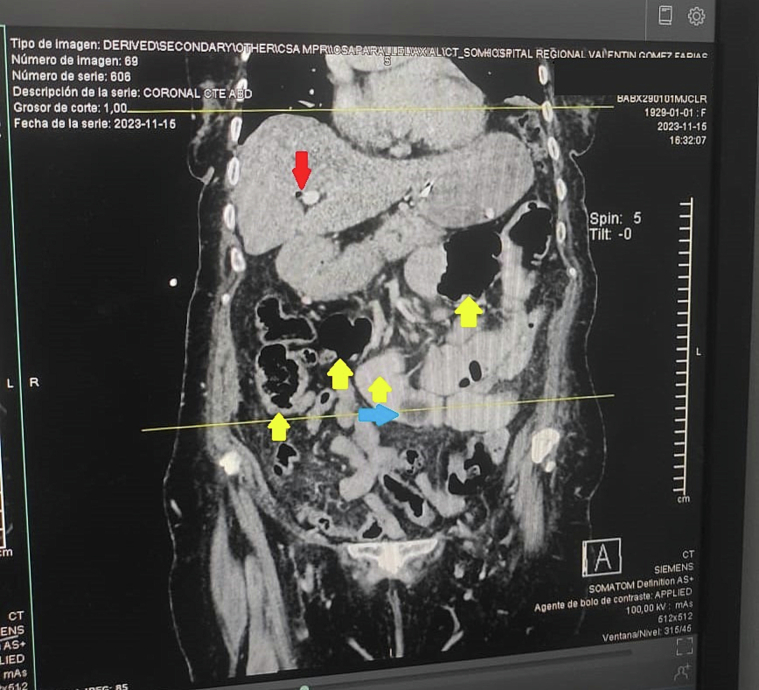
Shows a coronal view of a computed tomography scan of the abdomen, revealing multiple distended intestinal loops, suggestive of an intestinal obstruction. Additionally, pneumobilia is clearly visible, which is a key finding in the diagnosis of biliary ileus. These features are consistent with Rigler's triad, which includes intestinal obstruction, pneumobilia, and the presence of an ectopic gallstone. Red Arrow: Indicates pneumobilia, which is the presence of air within the biliary tract. Air should not be present in this location unless the patient has undergone an endoscopic procedure, such as an ERCP, or has a history of prior surgery. The presence of air suggests a communication between the biliary system and a segment of the intestine, allowing intestinal air to enter the biliary ducts. Blue Arrow: Highlights the density of the gallstone causing intestinal obstruction. Yellow Arrows: Represent the intestinal obstruction located proximally to the gallstone causing the blockage. (For interpretation of the references to colour in this figure legend, the reader is referred to the web version of this article.)
